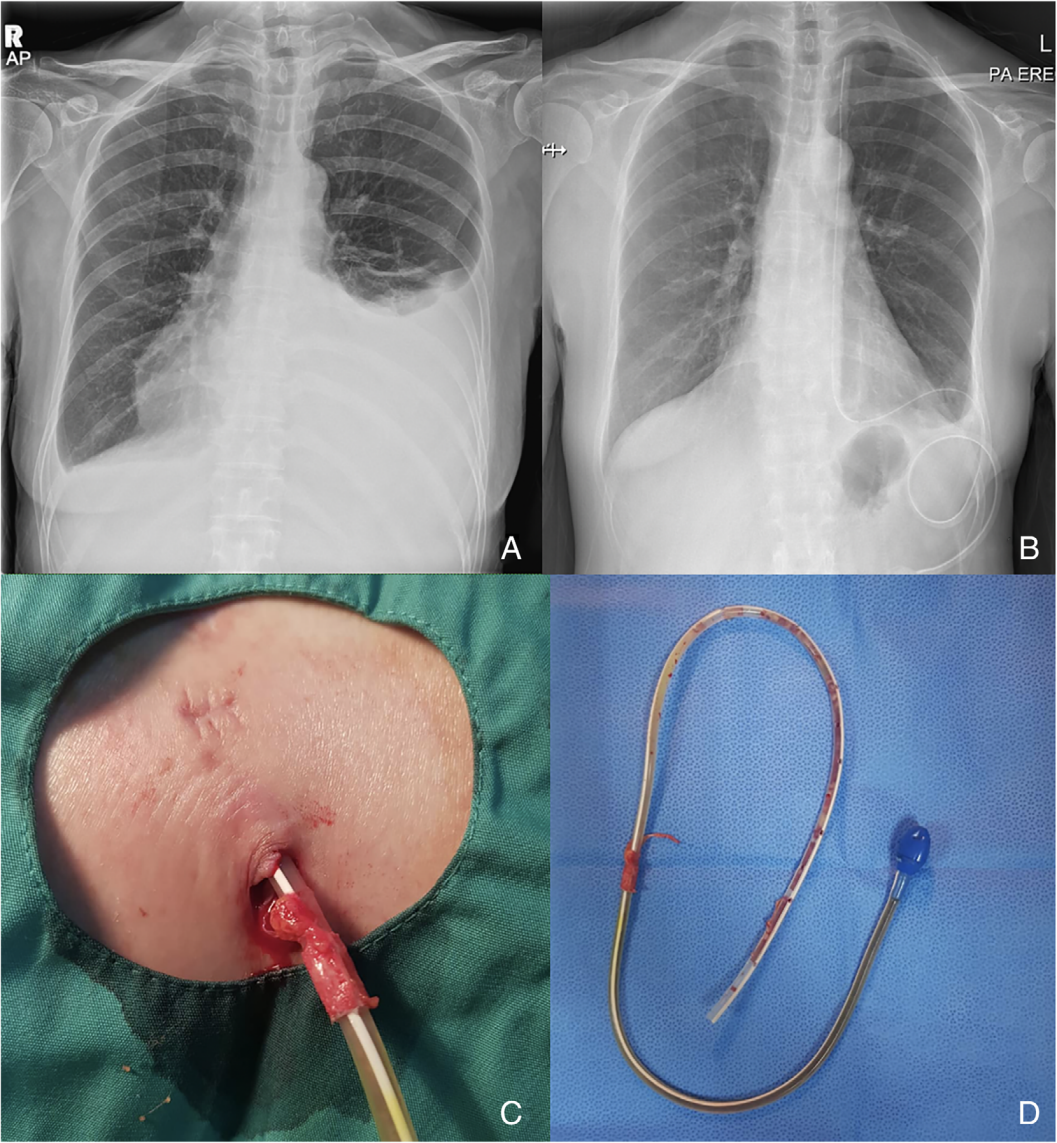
(A) Chest radiograph on admission showed moderate left pleural effusion. (B) Resolution of left pleural effusion with IPC insertion in situ at three months. (C) Removal of indwelling pleural catheter (IPC) with fibrous tissue engulfing the cuff. (D) Post‐removal intact IPC.
